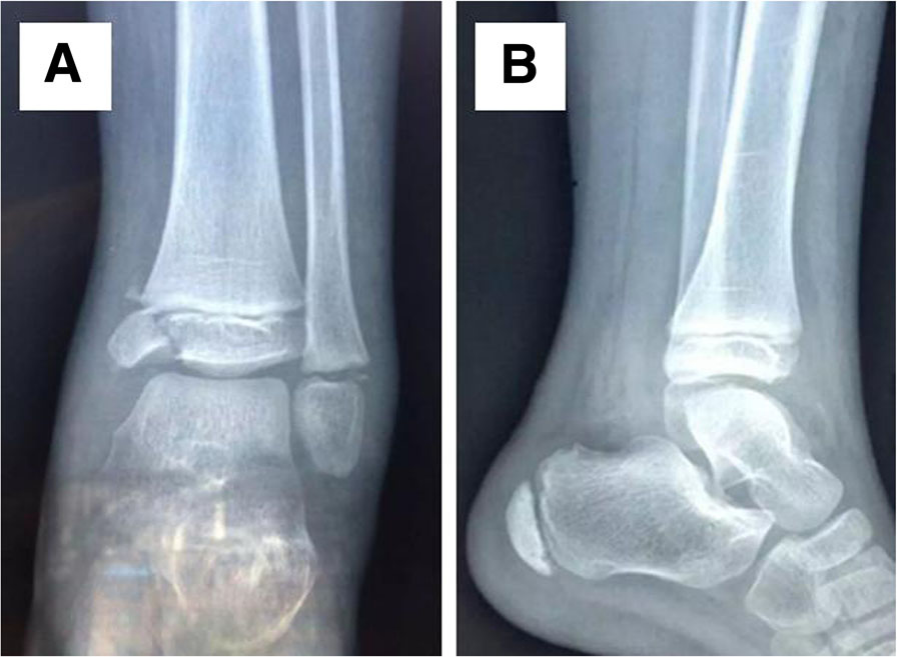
Preoperative X-rays (a, the anteroposterior film, b, the lateral film) of a 5-year-old boy with medial malleolus epiphyseal fracture (Salter-Harris III) and lateral malleolus epiphyseal fracture (Salter-Harris I) in right ankle joint
